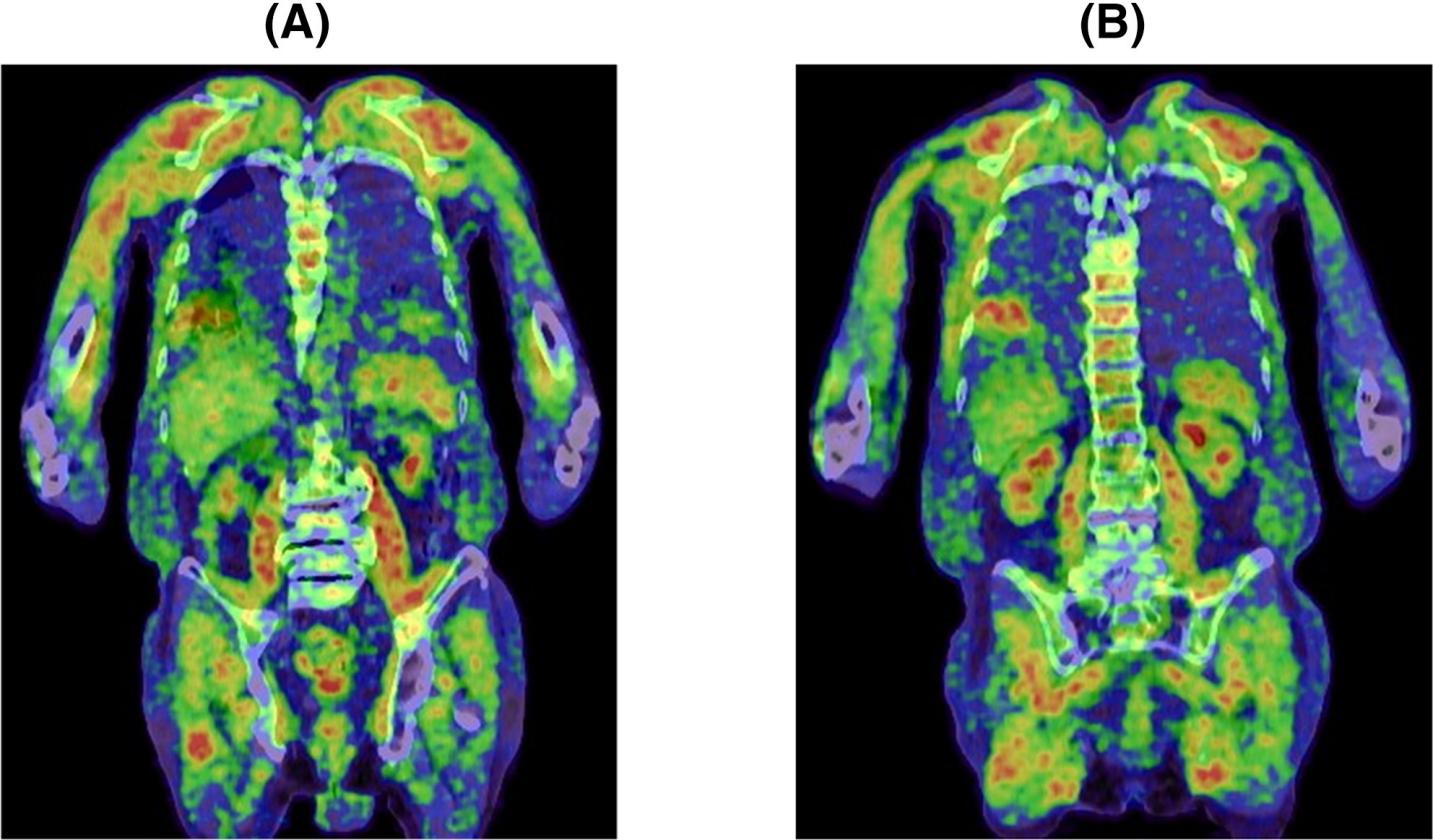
PET‐CT imaging findings on day 1 of hospitalization. Widespread accumulation centered on (A) soft shoulder tissues and on (B) quadriceps muscle. Blood glucose levels are normal. PET‐CT, positron emission tomography‐computed tomography.
